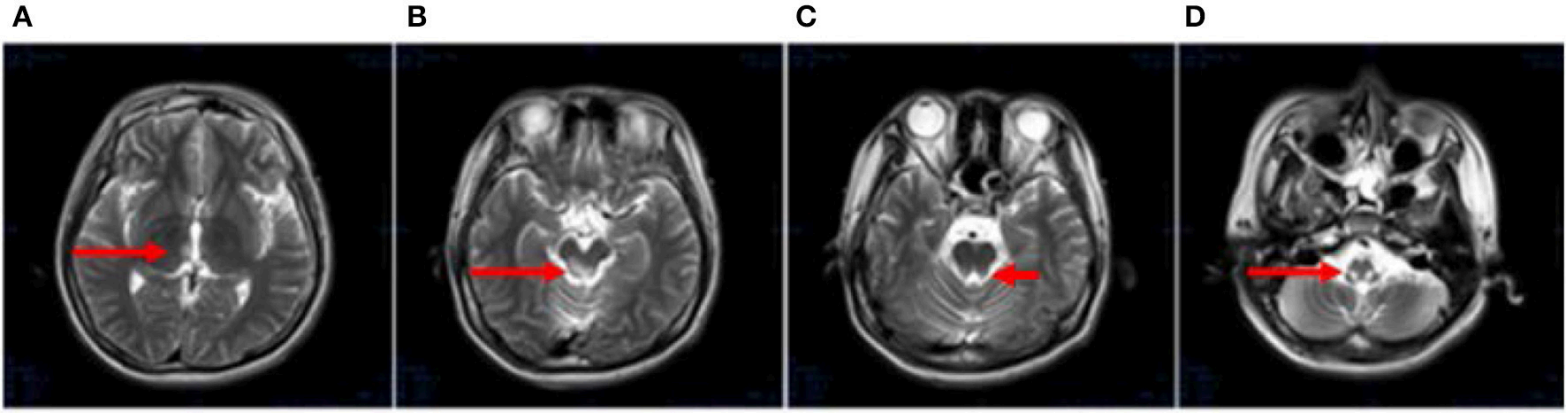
(A–D) Axial, T2-weighted MRI at 1 month following hospitalization revealed high signal intensity in the lateral ventricle, periaqueductal gray matter (bilateral hypothalamus and midbrain tegmentum, arrow in A,B), and medullary tegmentum (arrow in D). There were no evident lesions in the tegmentum of pons at this stage (small arrow in C).
